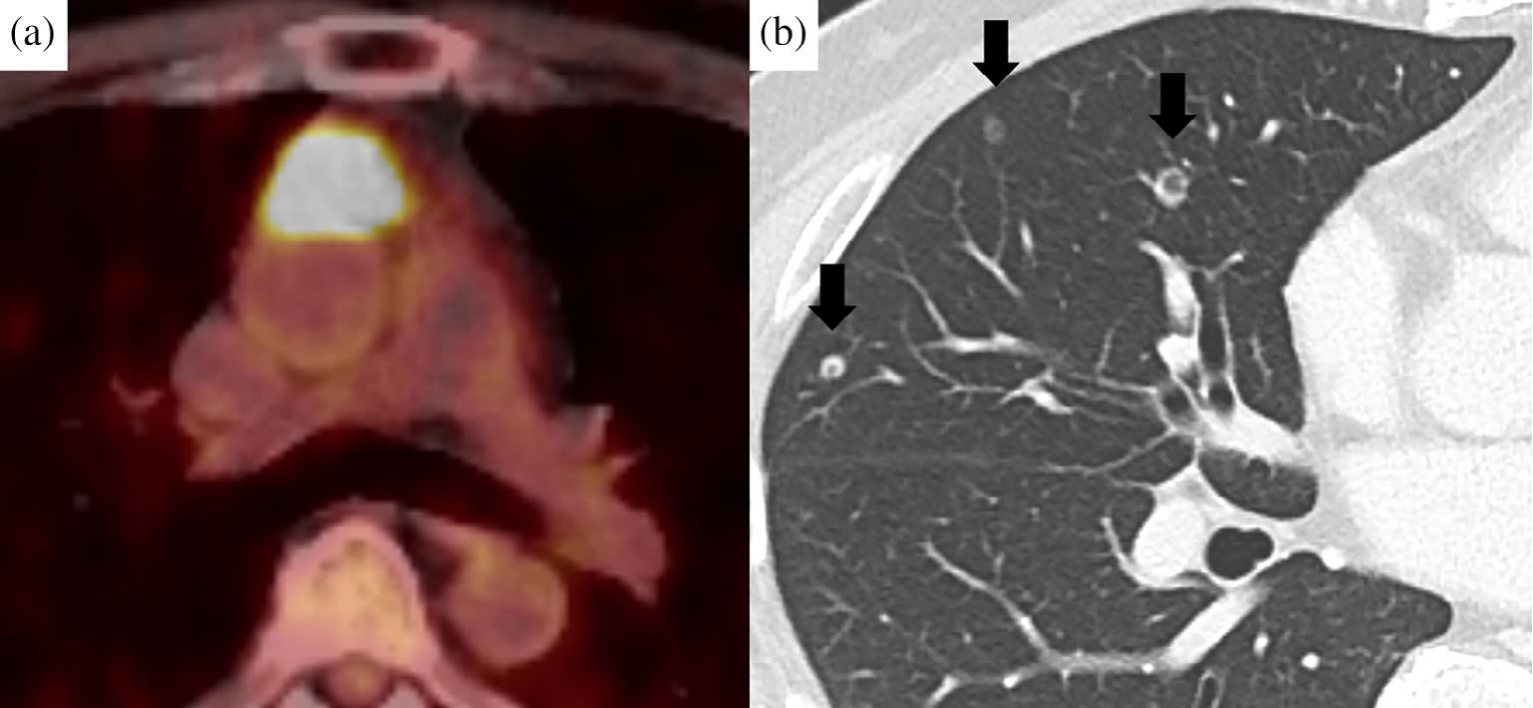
Images at 13 years after the initial examination. (a) Positron emission tomography‐computed tomography imaging showing strong accumulation of fluorodeoxyglucose in the anterior mediastinal tumor. (b) The bilateral lung nodules were suspected to be metastatic.
